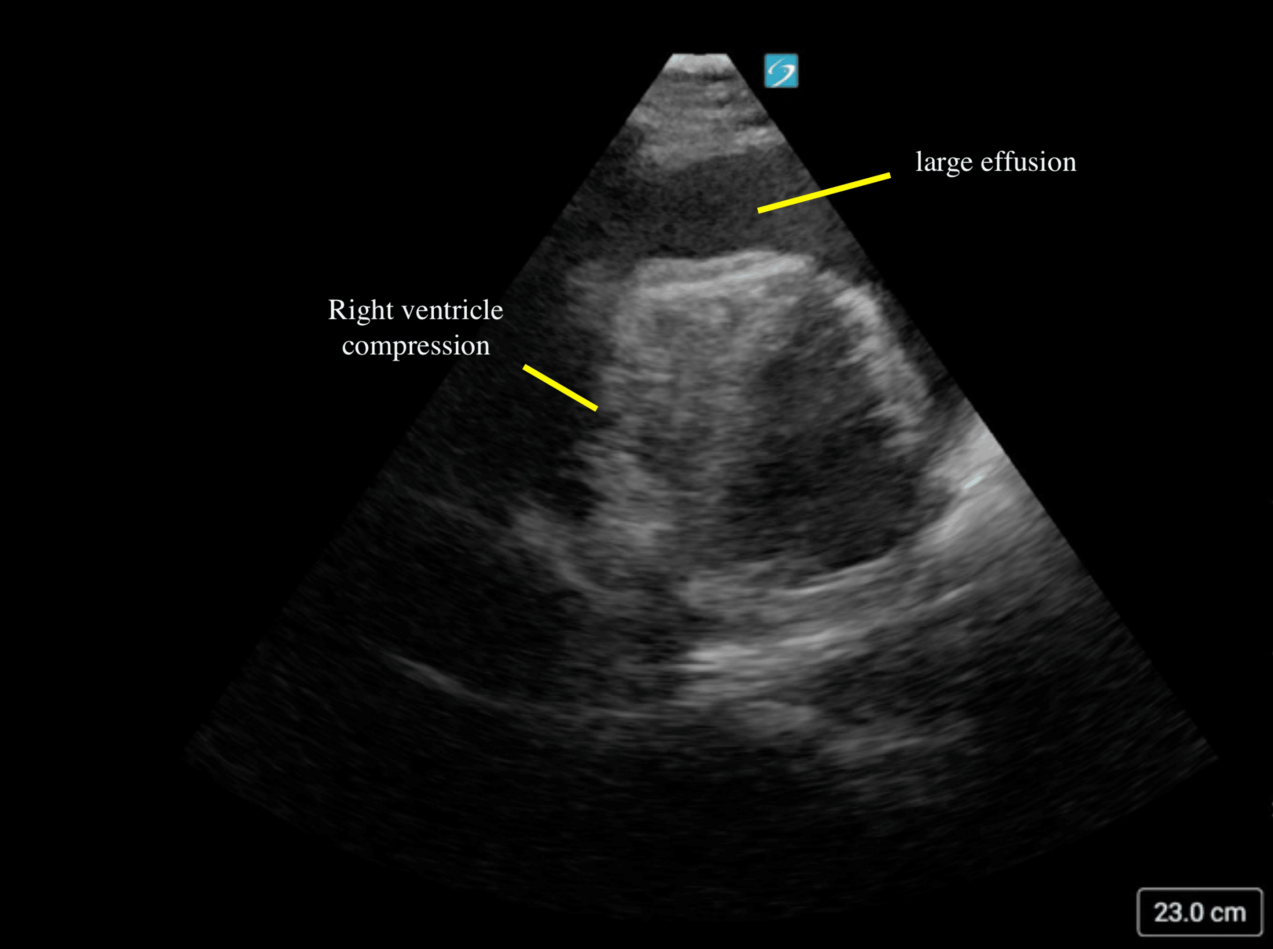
Apical four-chamber tamponade with right ventricular compression In this image, a large circumferential pericardial effusion is again noted. Compression of the right ventricle is also noted.
